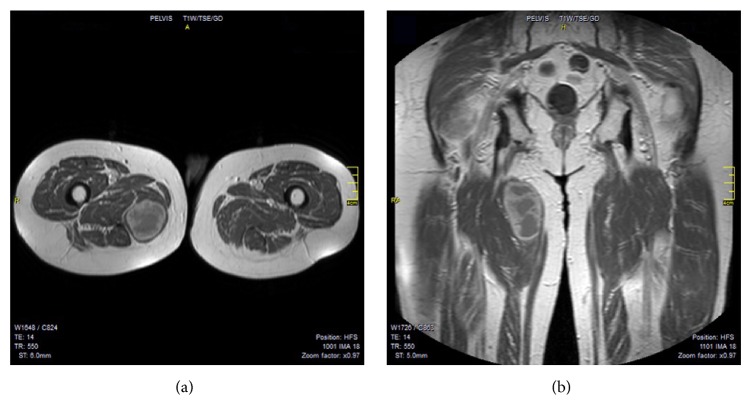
Axial (a) and coronal (b) T1 views of an MRI of the lesion in the context of the right adductor magnus muscle, with sparse areas of necrosis and irregular contrast enhancement.
